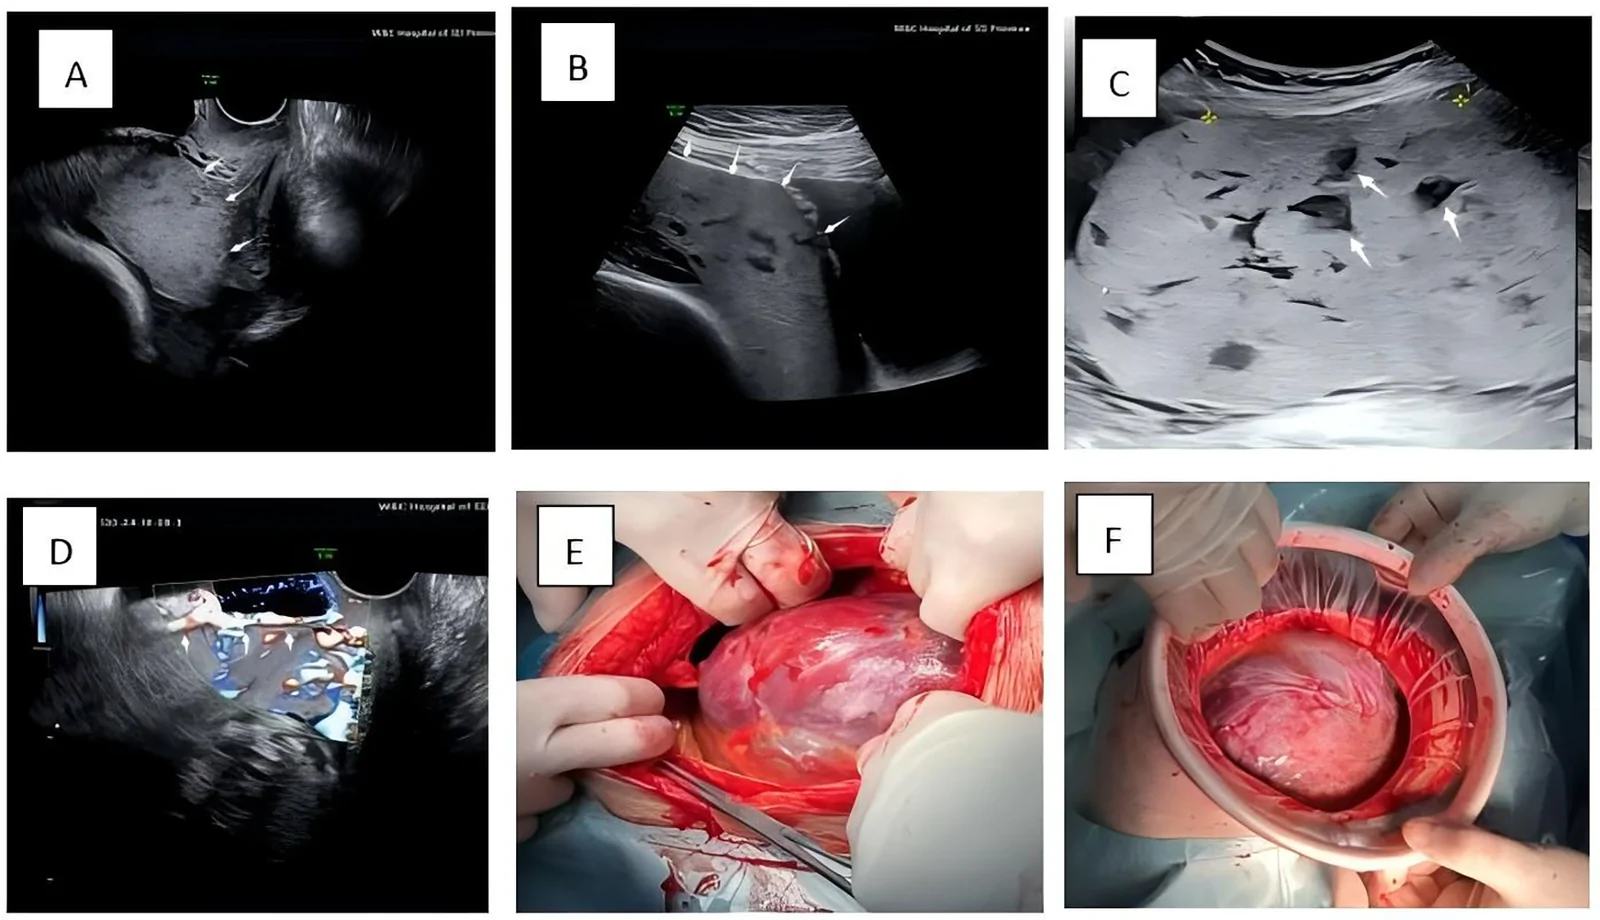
Typical ultrasound signs and intraoperative images. (A) Completely placental previa. (B) Disappearance of the retroplacental clear zone. (C) Placental lacunae. (D) Bridging vessels at the placental basal plate. (E, F) Intraoperative manifestations of placenta percreta. Placental tissue was observed to penetrate through the uterine serosa.
